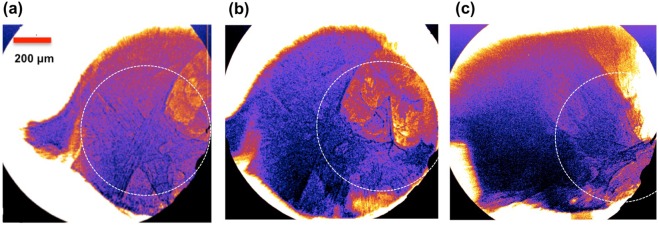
Blurring of LWFA X-ray images due to finite betatron emission length. Three LWFA phase contrast images of the Al-Si sample are shown. In (a,b) the sample is at the same orientation perpendicular to the laser axis. In image (b) the sample has been translated horizontally by approximately 30 *μ*m. In (c) the sample has been rotated by 90 degrees about the vertical axis. Regions of sharpest resolution are circled with a dotted line, with a radius of approximately 600 *μ*m at highest focus. In all images, blurring can be observed on the order of a millimeter away from the central point due to the emission length of the betatron source. Highest resolution imaging is obtained along the axis of the electron beam; only this section of the image is used for resolution analysis. Blurring due to the emission length of the X-ray source is not unique to betatron sources, also occurring with conventional synchrotron beams, but is exacerbated by high magnification in cases where the full beam is used for imaging.
